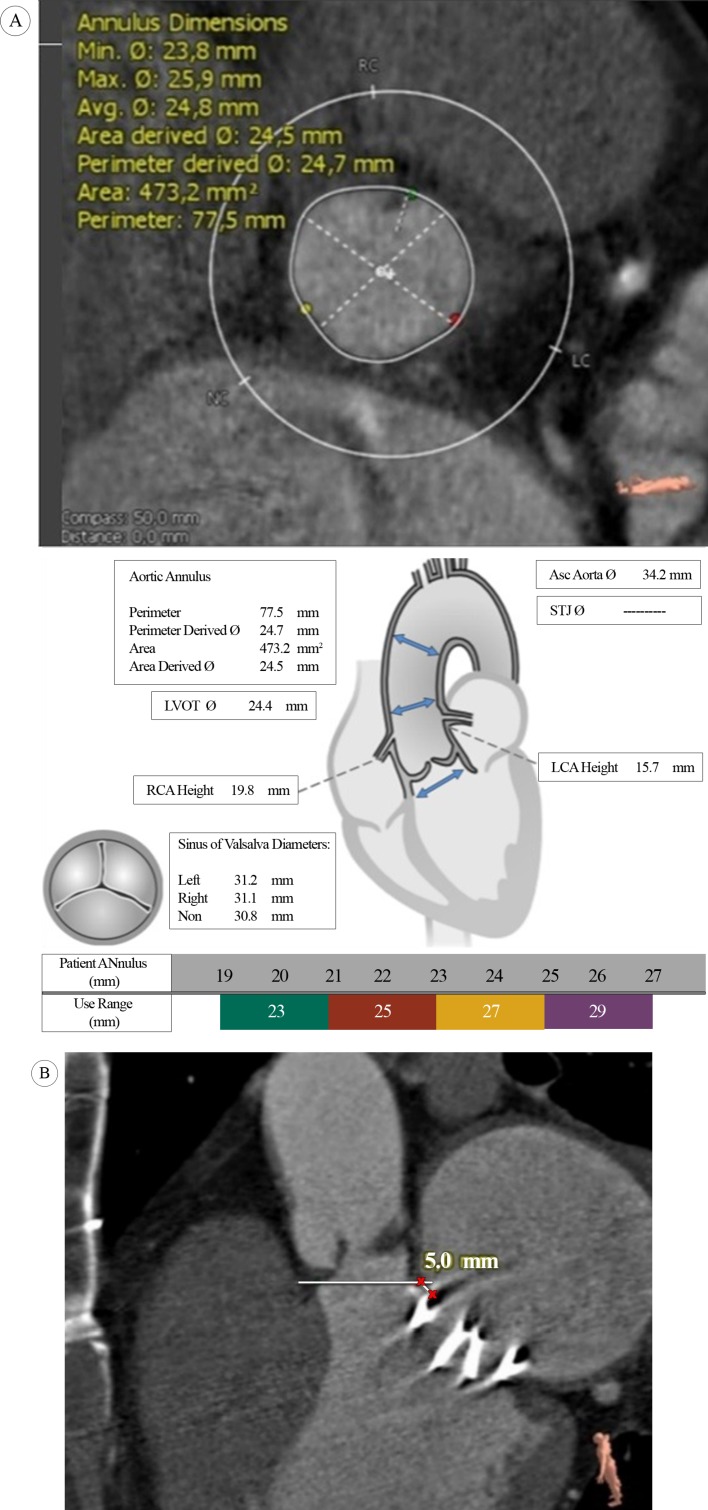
A) Computed tomography images of a calcified aortic valve and aortic annulus with valve sizes; and B) minimum distance between the prosthetic mitral valve and the aortic valve annulus
